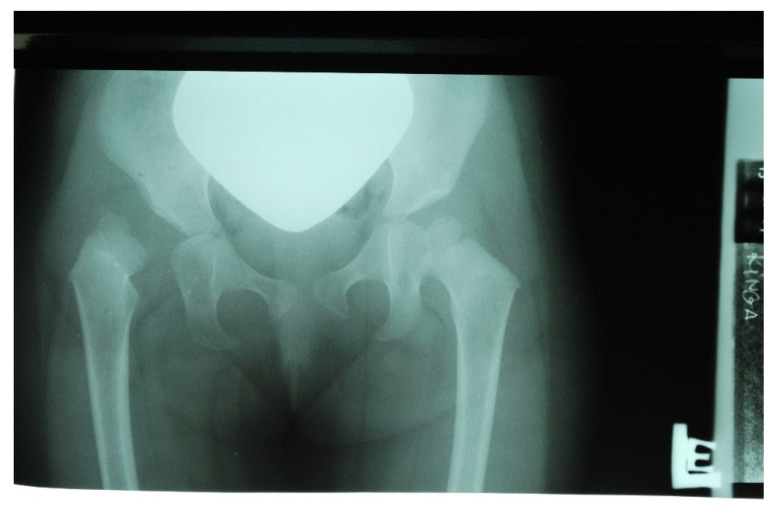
X-ray scan showing right hip dislocation in the girl (at 2 years of age).
